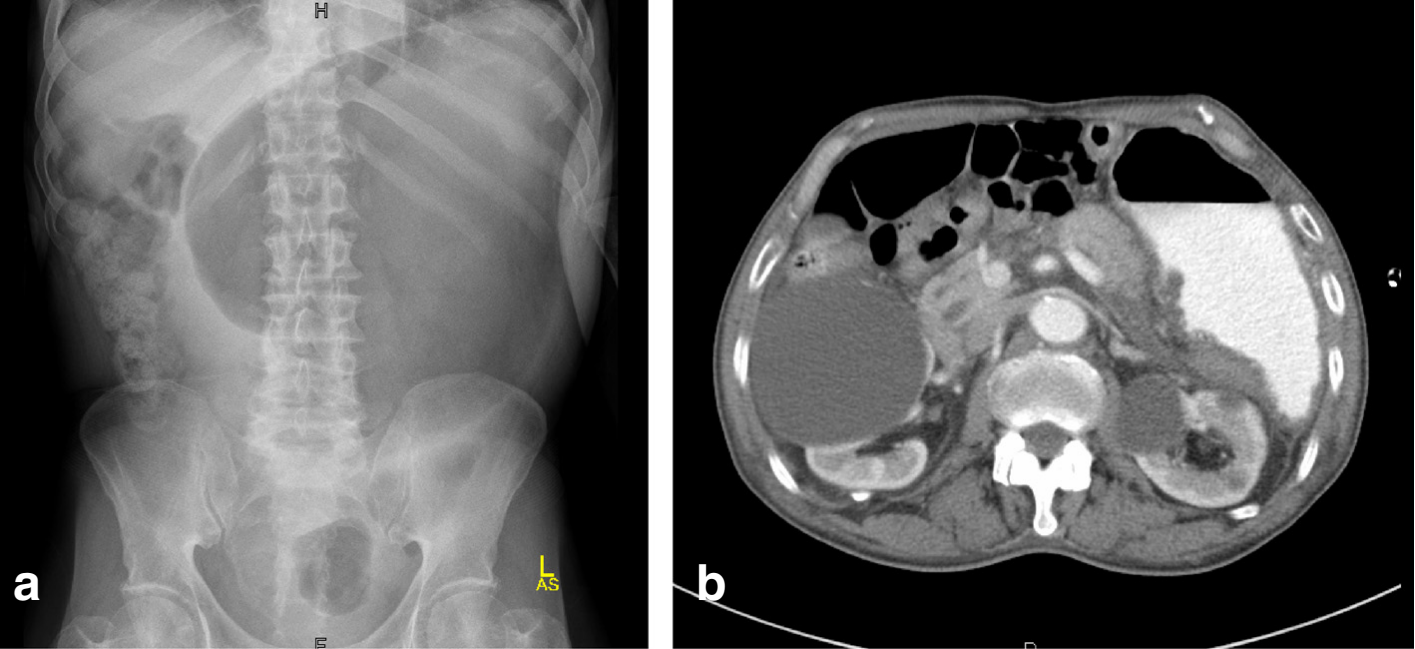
Radiologic findings. a. Plain abdominal X-ray showing a markedly dilated stomach b. Contrast CT-scan showing a significantly distended stomach with thickened wall and abnormal configuration. Note also air-fluid level.
